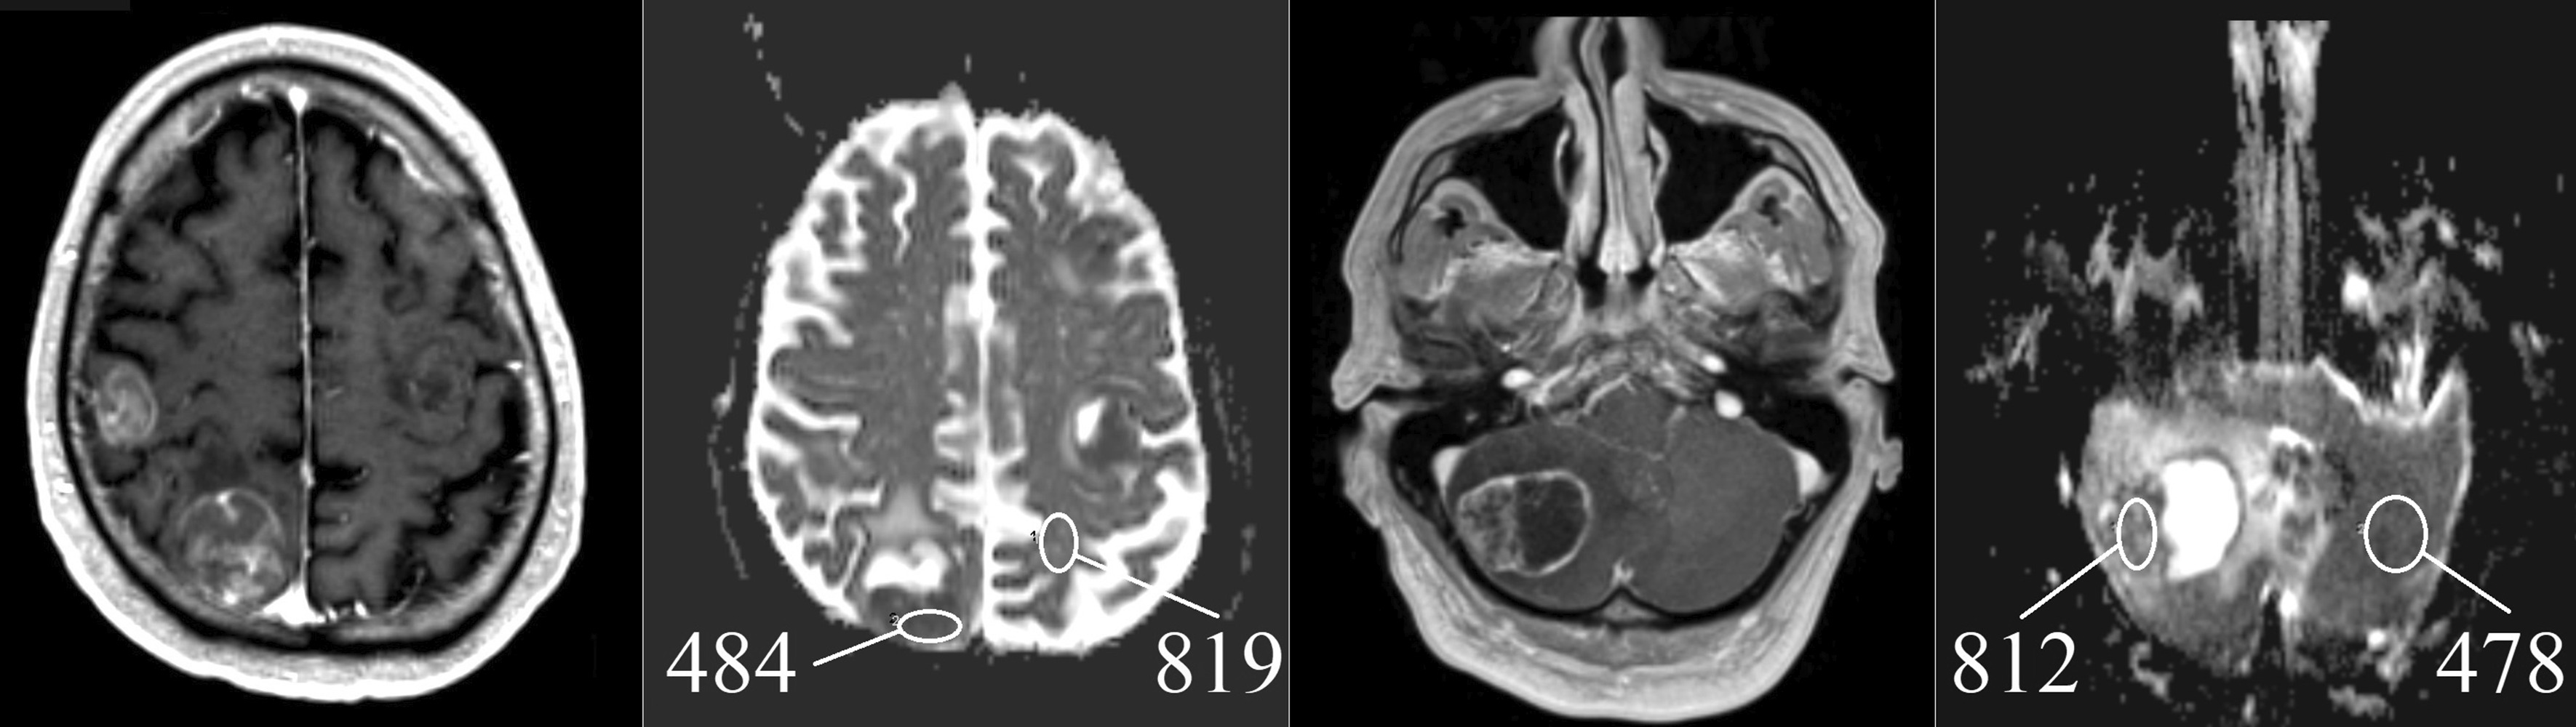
Two examples of ADC-ratios of brain metastasis in 3-T MRI (T1 with contrast agent and ADC map): left: typical SCLC with ADC ratio of 484/819 = 0.59, right: NSCLC with ADC ratio of 812/478 = 1.70. *SCLC* small cell lung cancer, *NSCLC* non-small cell lung cancer, *ADC* apparent diffusion coefficient
